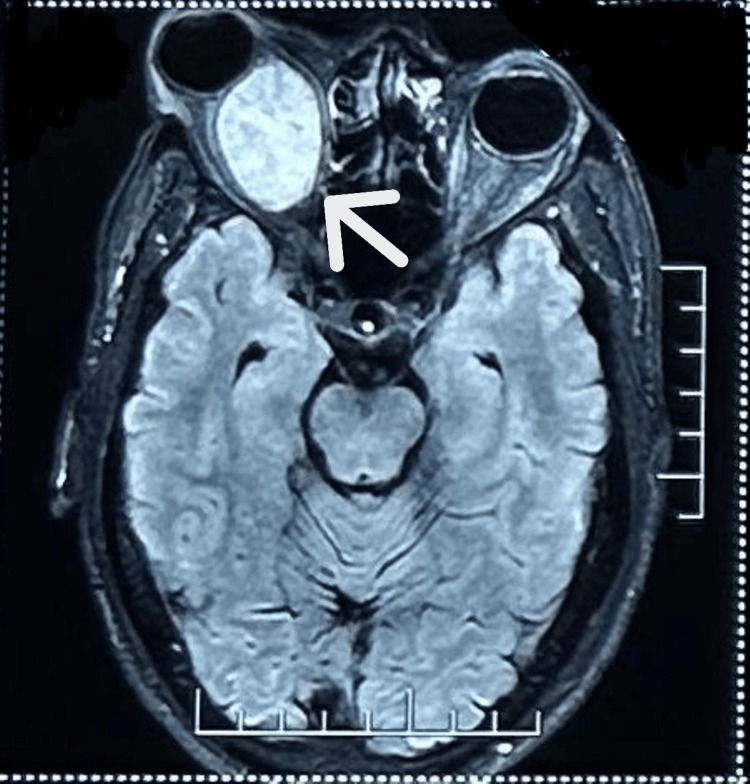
Magnetic resonance imaging of the brain and orbits revealing a well-defined altered lesion measuring 3.2 × 2.4 × 3.4 cm in the intraconal compartment of the right retrobulbar region.
